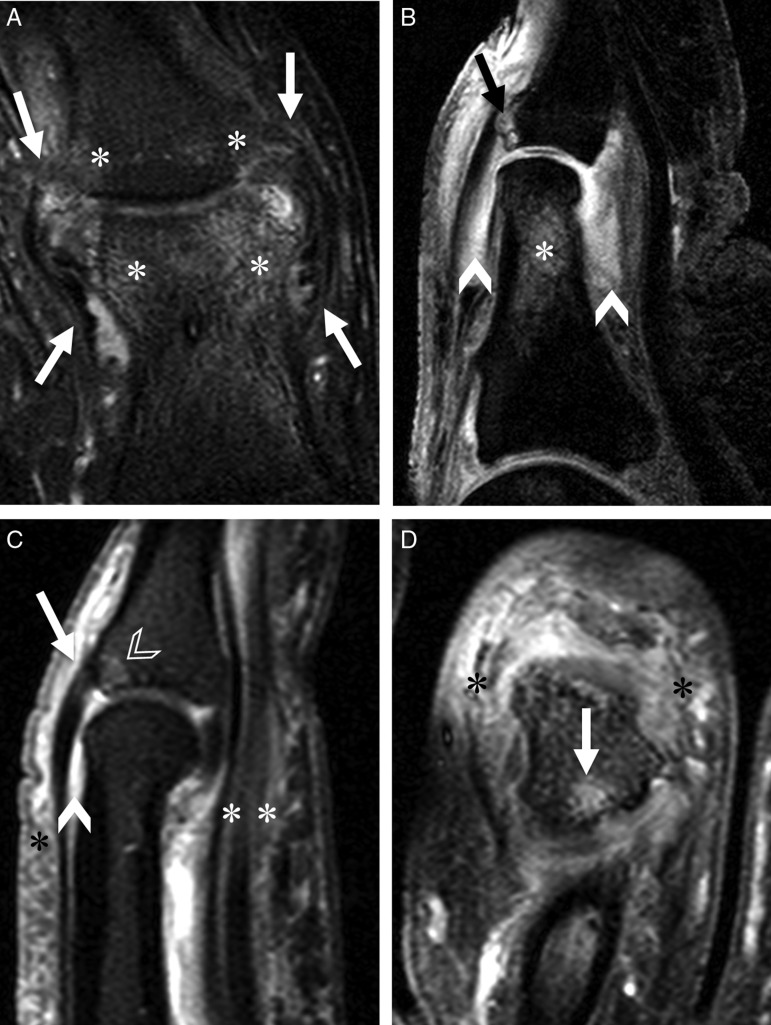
Enthesitis and osteitis in dactylitis. Fat-saturated contrast-enhanced T1-weighted images. (A) Coronal image of the left big toe of a 37-year-old man. Bilateral collateral ligament insertions and origins show mild to moderate enhancement (arrows). Mild to moderate bone marrow enhancement adjacent to the origins and insertions of the ligaments can also be seen (asterisks). (B) Sagittal image of the left big toe of same patient as (A) showing focal bone marrow enhancement at the extensor tendon insertion (arrow) and diffuse enhanced bone marrow (asterisk). Intracapsular synovium of the interphalangeal joint was swollen and moderately enhanced (arrowheads). (C) Sagittal image of the right middle finger of a 41-year-old man. The extensor tendon insertion demonstrated mild enhancement (arrow) with associated bone oedema (white outlined arrowhead). Diffuse enhancement of the flexor tenosynovium (white asterisks), extracapsular soft tissues (black asterisk), and moderate enhanced intracapsular synovium (arrowhead) in the proximal interphalangeal joint were also seen. (D) Coronal image of the right 2nd toe of a 42-year-old woman. The base of the middle phalangeal bone was partially enhanced (arrow), adjacent to the extensor tendon insertion. Diffuse soft tissue enhancement was also shown (black asterisks).
